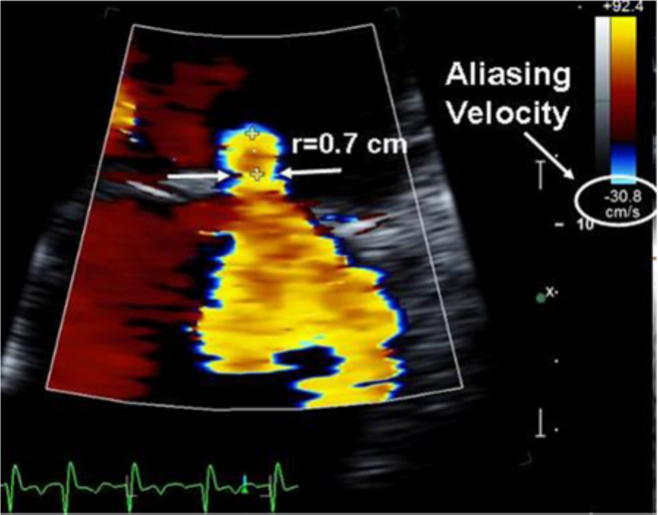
Calculation of EROA by PISA method. In this apical four chamber view, the PISA region is displayed from a magnified apical view, and the hemispheric PISA radius R is shown between the crosshairs. Note the change of the color Doppler scale, with a baseline shift in the direction of the MR jet (e.g. “down” in this example). The aliasing velocity is 30.8 cm/s. EROA can be calculated as the product of 2πR^2^ x Aliasing Velocity ÷ Peak Velocity of MR (peak velocity not shown). The vena contracta is indicated as the distance between the two arrows. EROA = effective regurgitant orifice area; PISA = proximal isovelocity surface area.
